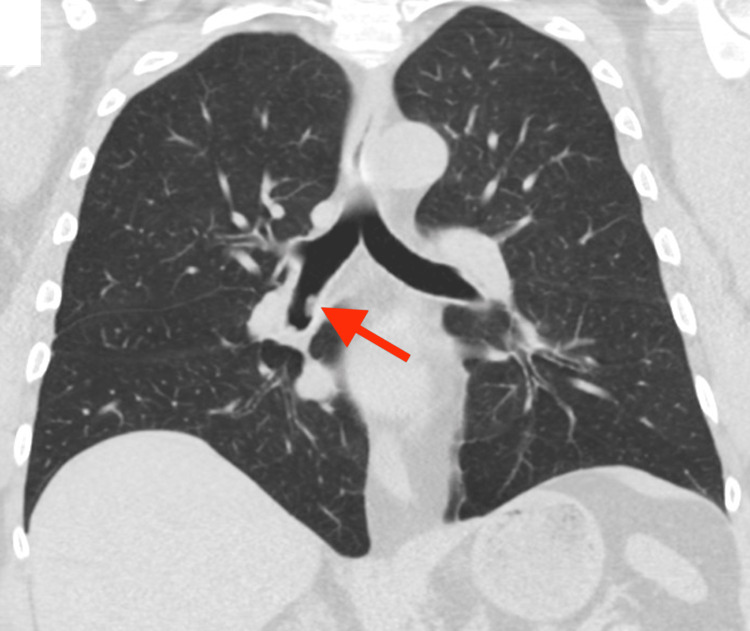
A coronal image of the follow-up chest CT without intravenous contrast demonstrating the interval removal of the endobronchial foreign body with a subcentimeter-sized residual soft nodule within the bronchus intermedius (red arrow).
